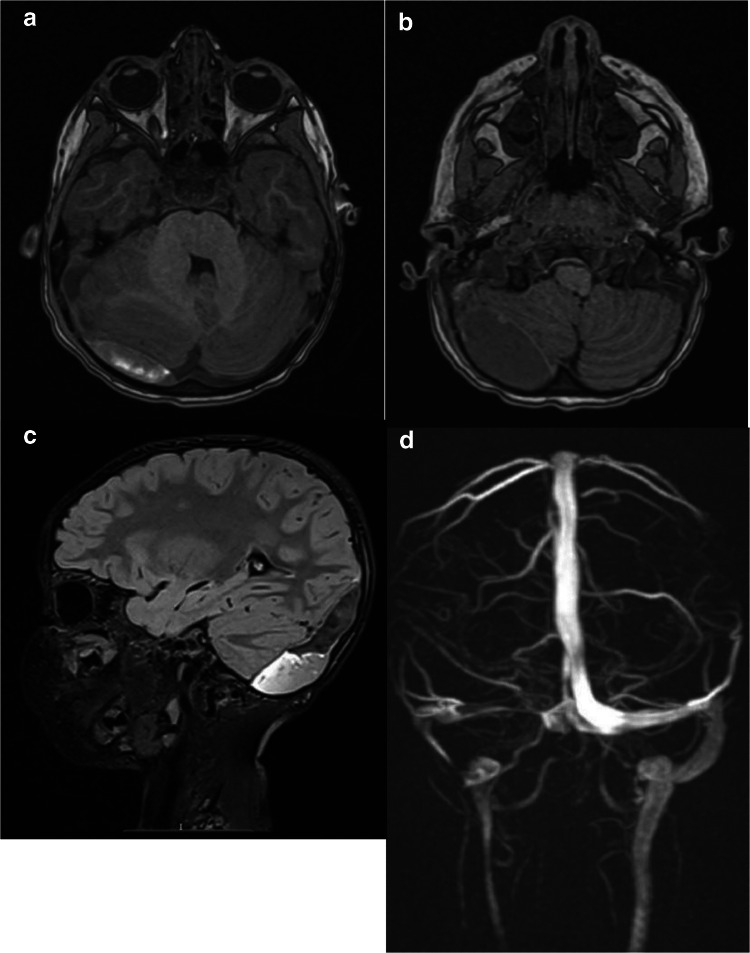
a and b T1-weighted axial and c T2-weighted SPACE Dark Fluid sagittal non-contrast enhanced MR images of extradural haematoma with local mass effect (4 mm midline shift to the left) and extrinsic compression of the right transverse sinus. d MRV representation of right sigmoid sinus thrombus resulting in near-total occlusion of this venous segment.
